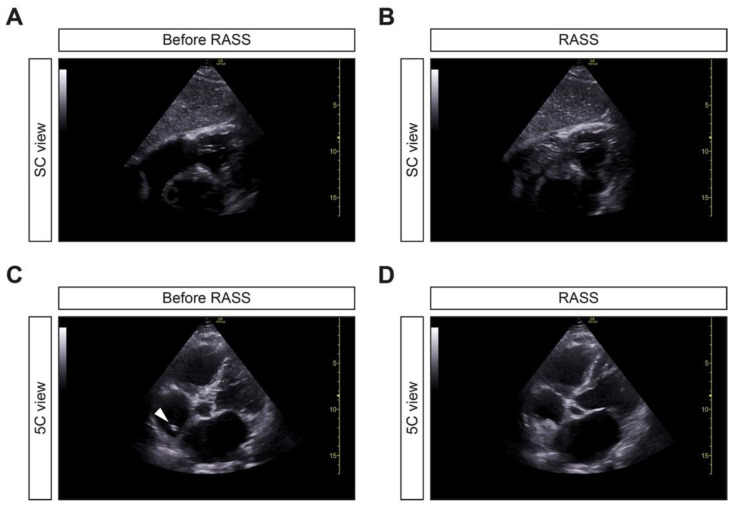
Application of the RASS for US-guided tip positioning of retrograde-tunneled HDCs. (A,B) After placement of the retrograde-tunneled HDC, focused echocardiography using a SC view to visualize the right atrium and RASS was performed. If the RASS appeared immediately, within one second, HDC tip positioning was considered to be adequate. (C,D) The HDC positioning was considered to be in the adequate position if the tip was visualized within the right atrium (arrowhead), with reconfirmed immediate RASS visualization in the apical 5C view, within one second. Abbreviations: HDC, hemodialysis catheter; RASS, rapid atrial swirl sign; SC, subcostal; US, ultrasound; 5C, five-chamber.
